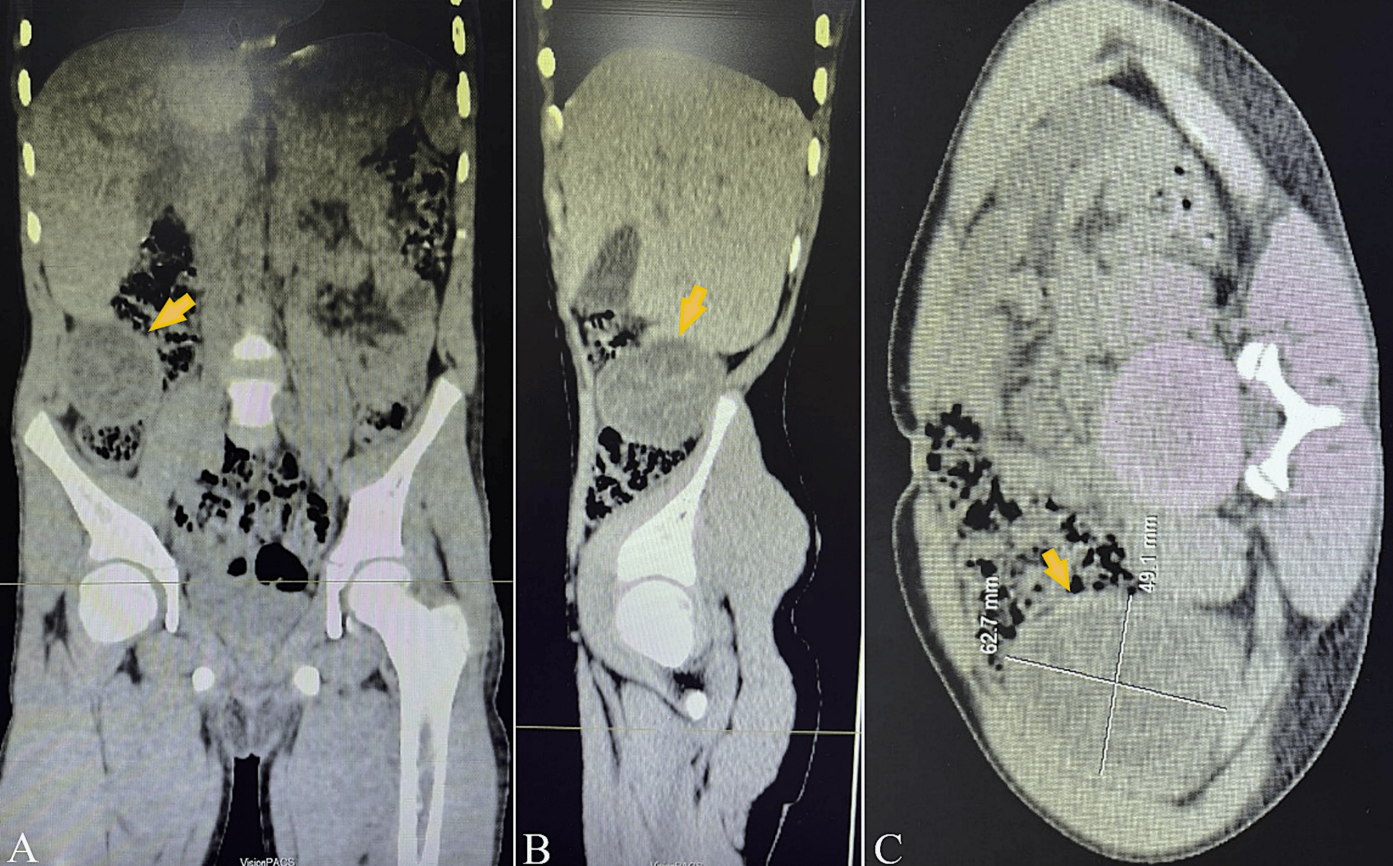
Non-contrast abdominal CT demonstrating a well-circumscribed right retroperitoneal cystic mass measuring 62.7 mm in maximal diameter on coronal (A), sagittal (B), and axial (C) images (arrows).
